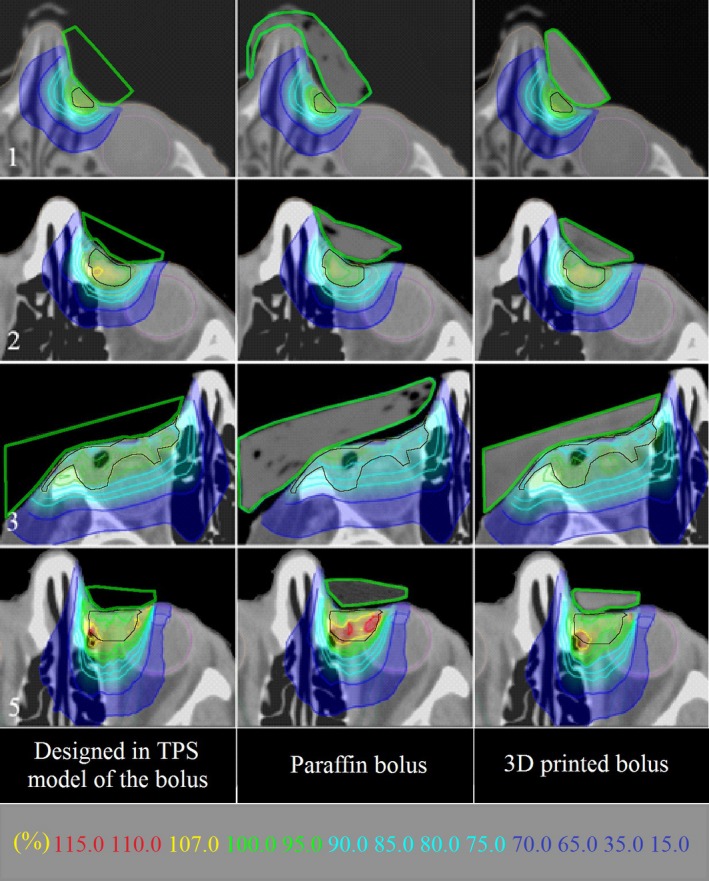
Comparison of dose distributions distal to reference model, paraffin, and 3D‐printed boluses and superimposed on transverse CT scans for select patients (1, 2, 3, 5). Bolus shapes are outlined in green. PTV is outlined in black. Note isodose values in key at bottom of figure.
